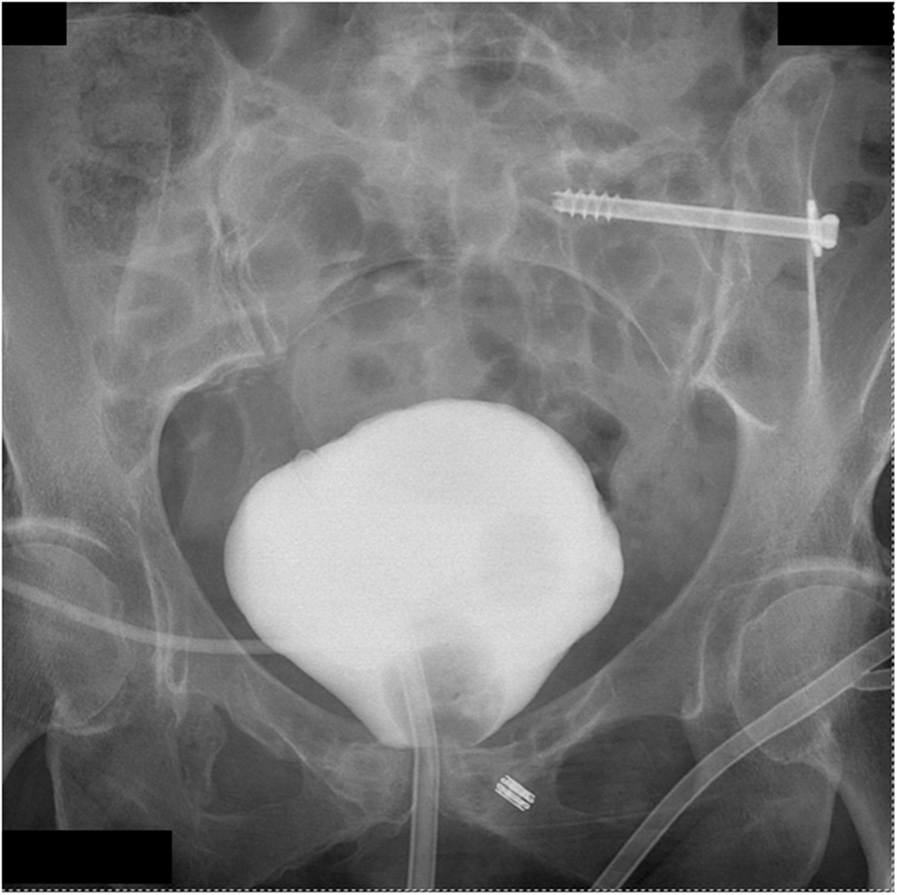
Cystography after hardware removal and bladder repair. AP pelvic x-ray taken during cystography after symphyseal hardware removal and bladder repair shows no bladder leak. The cystoscopy shows the two urinary catheters used during the operative repair
